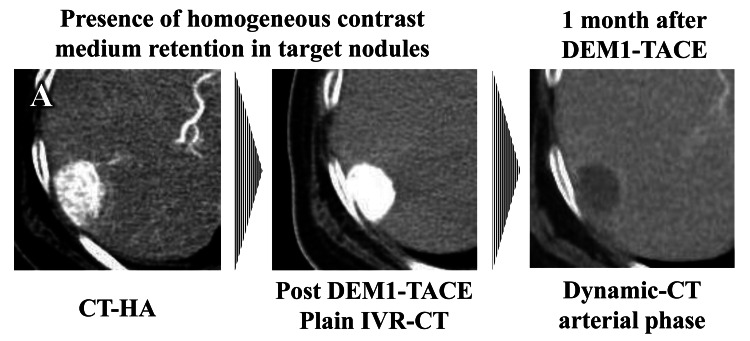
Typical treatment outcomes of homogeneous contrast medium retention patterns evaluated by IVR-CT in target nodules after DEM1-TACE CT-HA: computed tomography-during hepatic arteriography; DEM1-TACE: drug-eluting bead (DEB)-TACE using DC Bead M1^TM^; IVR-CT: interventional radiology-computed tomography; mRECIST: modified Response Evaluation Criteria in Solid Tumors (A) Target nodule showed complete response in mRECIST after DEM1-TACE
